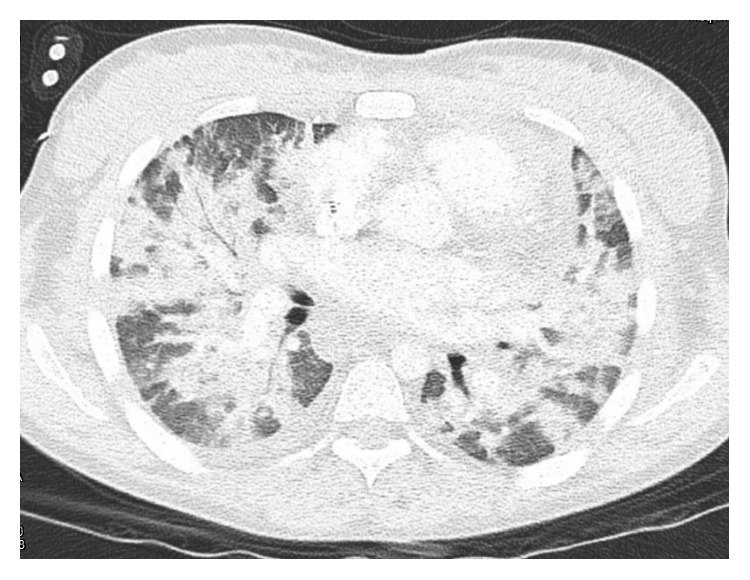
CTA of the chest with IV contrast reveals extensive bilateral consolidations.
